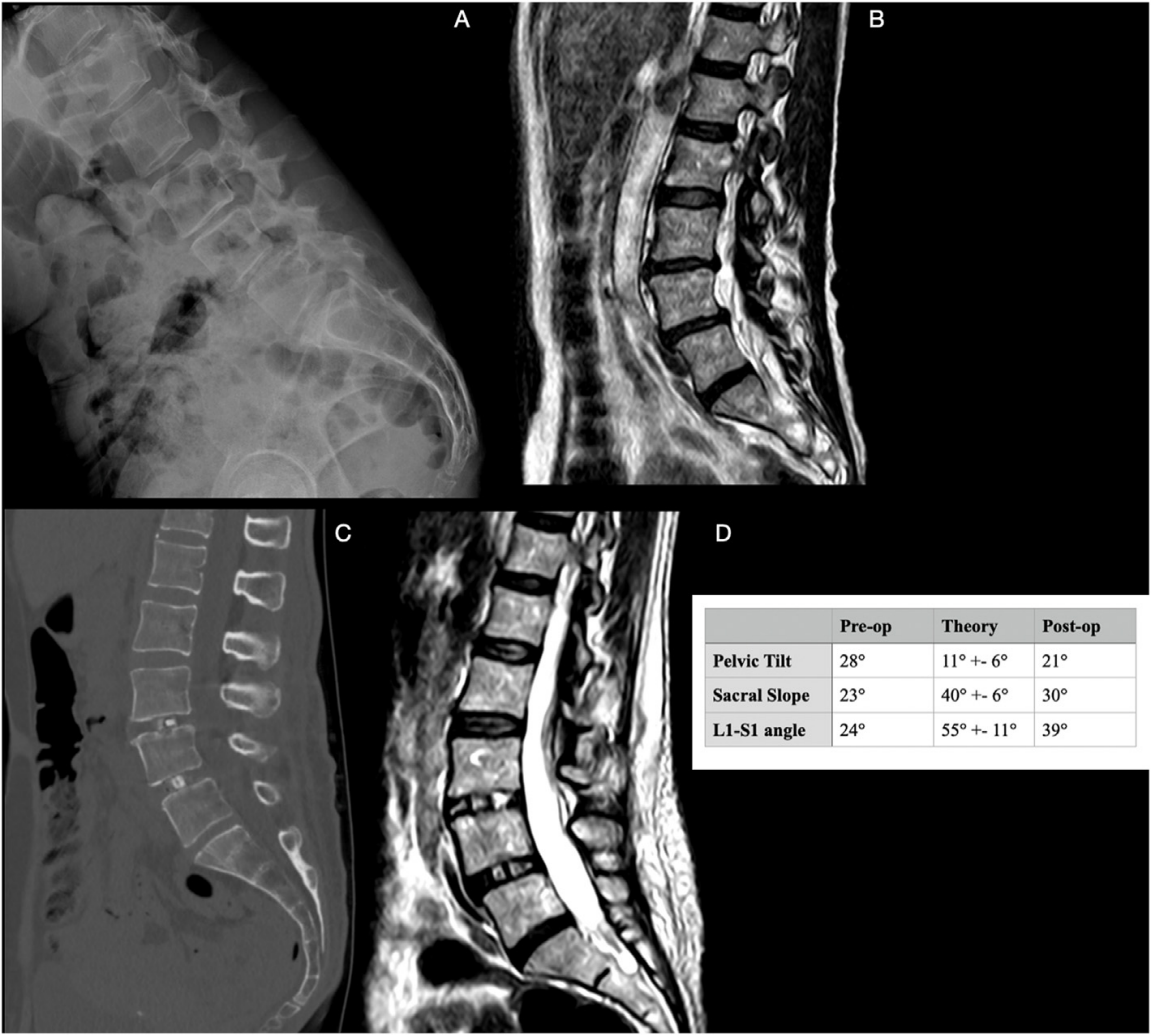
We report the clinical case of a 57-year-old woman with chronic low back pain and degenerative discopathy with L4-L5 listhesis (standing Rx A, and preoperative MRI, B) who benefited from a significant improvement in sagittal balance parameters after stabilization surgery with double MS-TLIF L3-L4-l5 with lordotic cages (CT scan after 1 month, C and MRI control after 6 months).
